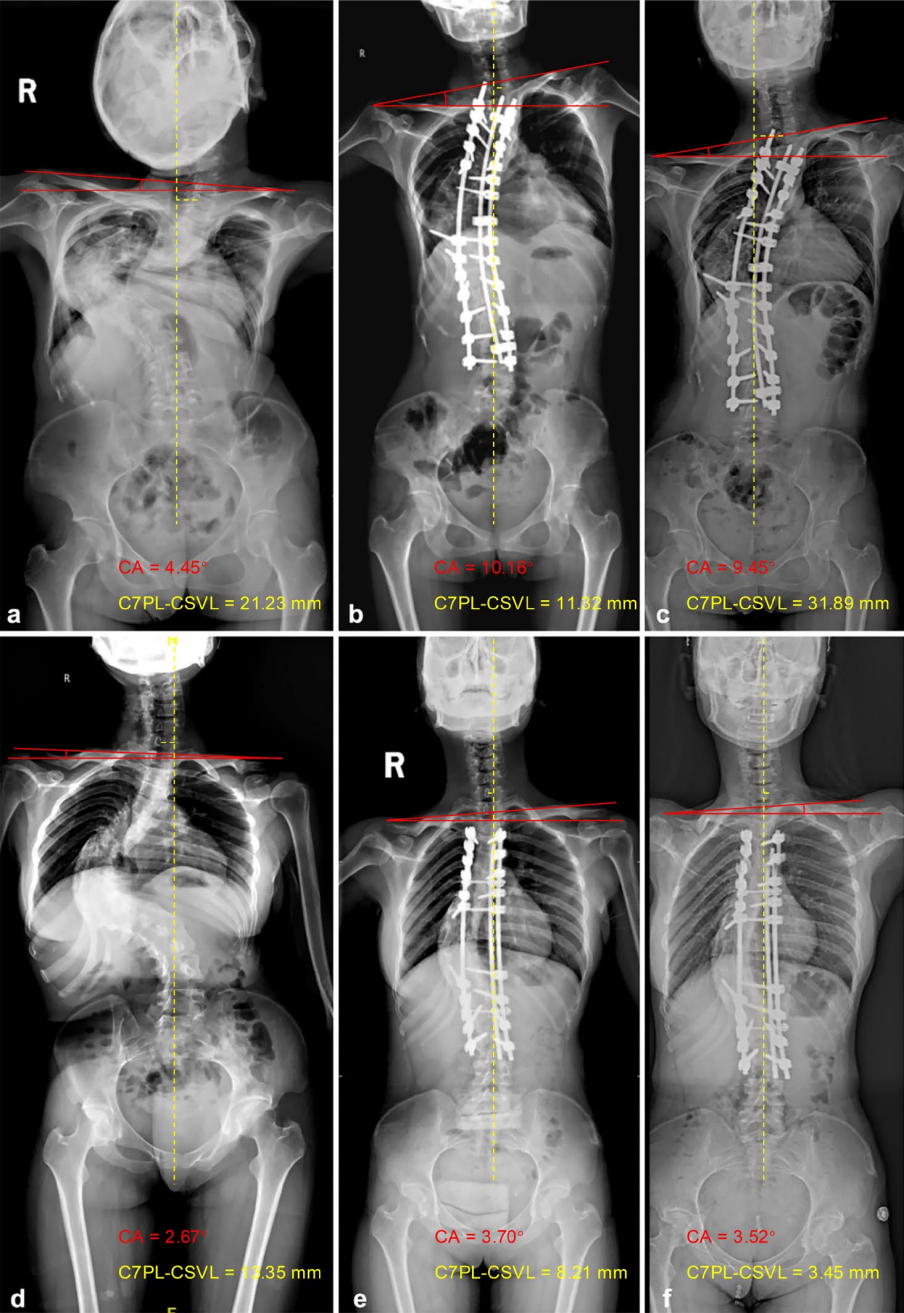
(a–c) The preoperative, immediately postoperative, and the last follow‐up radiographs of a 38‐year‐old female who was diagnosed with severe and rigid idiopathic scoliosis and underwent posterior instrumentation. The postoperative CA was 10.16° and the postoperative C7PL‐CSVL was 11.32 mm. Based on the predictive equation, the Risk Index of coronal imbalance was 15.02 (−5.277 + 1.922 × 10.16 + 0.068 × 11.32), which was greater than the cutoff value (3.15), indicating a high risk of coronal imbalance during the follow‐up. At the last follow‐up, coronal imbalance was observed with C7PL‐CSVL of 31.89 mm; (d–f) The preoperative, immediately postoperative, and the last follow‐up radiographs of a 26‐year‐old female who was diagnosed with severe and rigid congenital scoliosis and underwent posterior instrumentation. The postoperative CA was 3.70° and the postoperative C7PL‐CSVL was 8.21 mm. Based on the predictive equation, the Risk Index of coronal imbalance was 2.39 (−5.277 + 1.922 × 3.70 + 0.068 × 8.21), which was less than the cutoff value (3.15), indicating a low risk of coronal imbalance during the follow‐up. At the last follow‐up, coronal balance was maintained well with C7PL‐CSVL of 3.45 mm.
